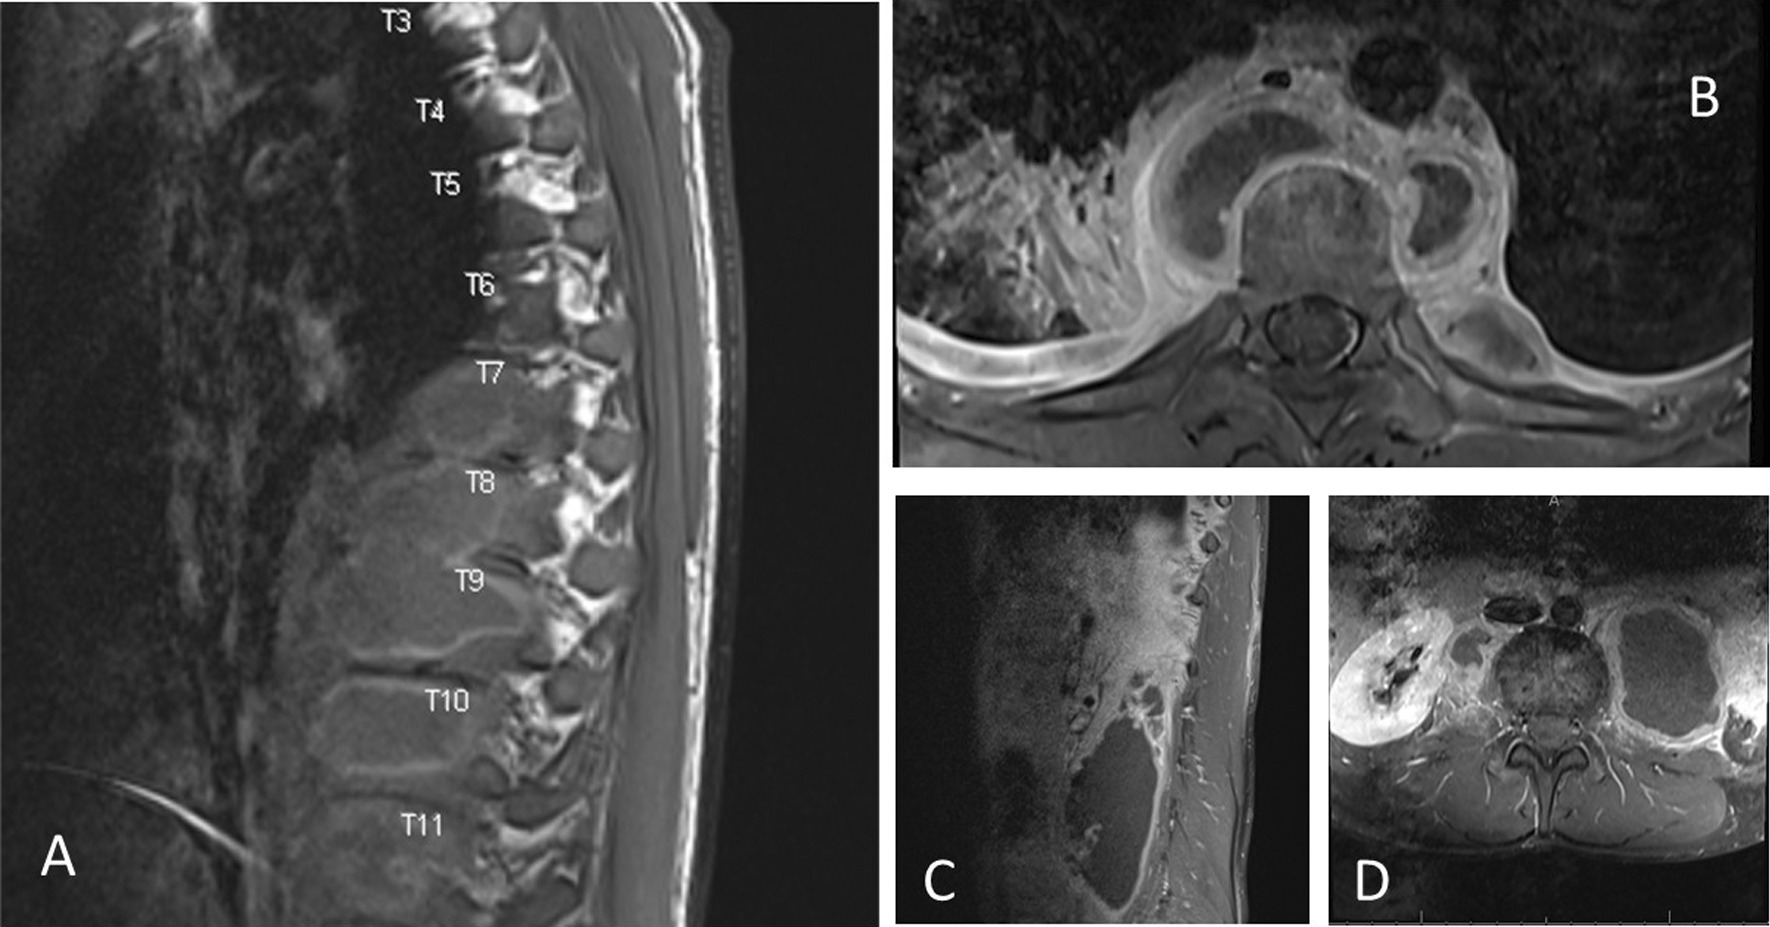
a, b MRI of the spine showing multi-level paraspinal collections, and psoas abscesses (c and d)
